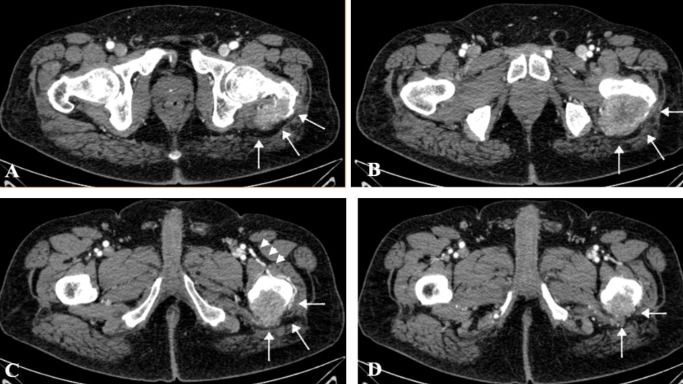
Angio-CT axial slice showing renal cell carcinoma metastasis at the femoral neck. (A) Tumor apex at the femoral neck (white arrows); (B) Proximal third of Bone Tumor (white arrows); (C) Distal third of Bone Tumor (white arrow) and arterial supply of the deep femoral artery (white arrowhead); (D) Bottom of the Bone Tumor.
